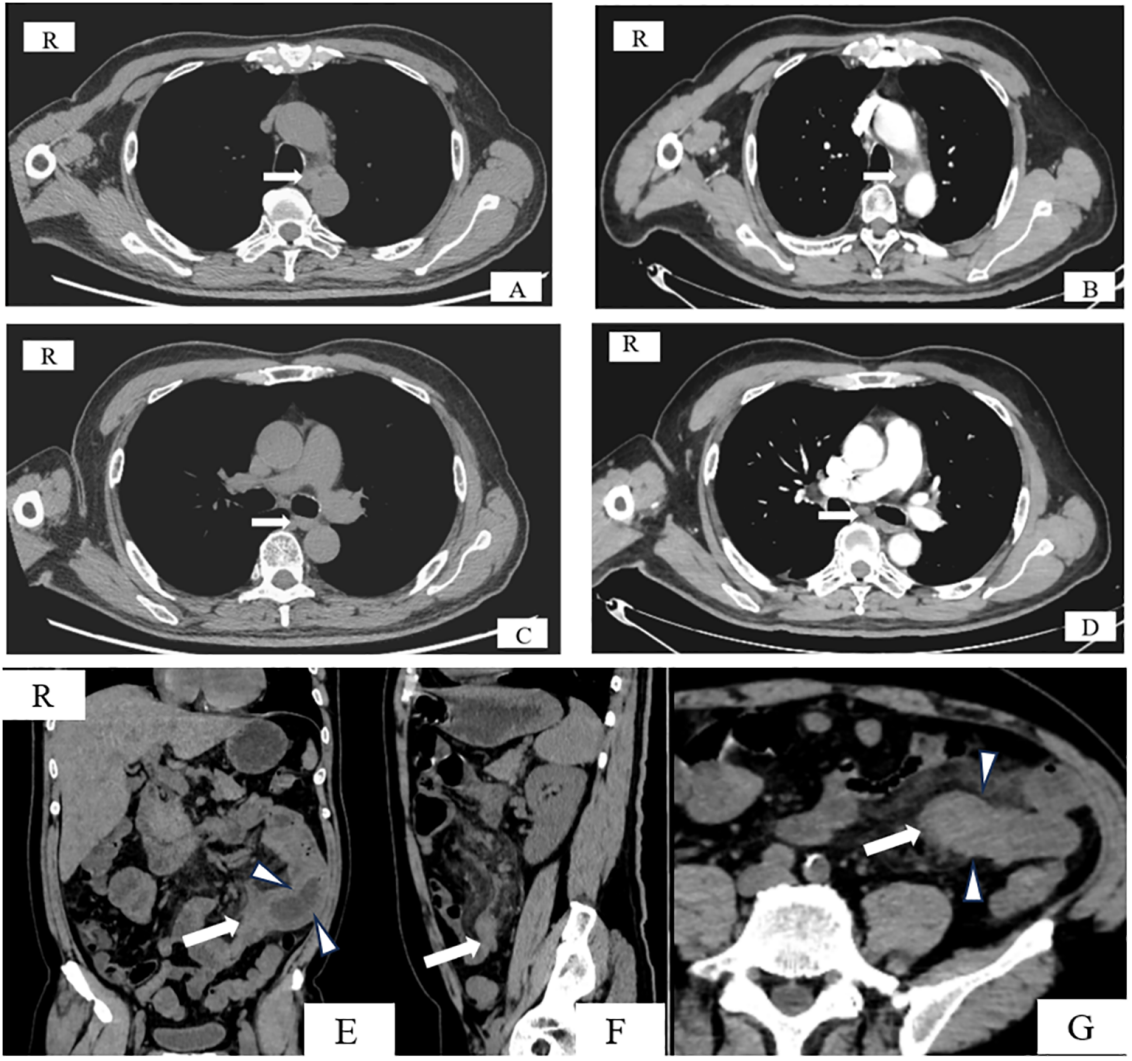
CT images. (A, B) Axial CT images of the esophageal primary tumor, 1 month preoperatively (A) and preoperatively (B), showing no significant changes. (C, D) Axial CT images of lymph nodes below the tracheal carina, 1 month preoperatively (C) and preoperatively (D), showing no significant changes. (E–G) Coronal, sagittal, and axial CT images demonstrating uneven thickening of the intestinal wall (short arrow), luminal narrowing, and occlusion, with an obstructive point visible in the distal portion (long arrow).
